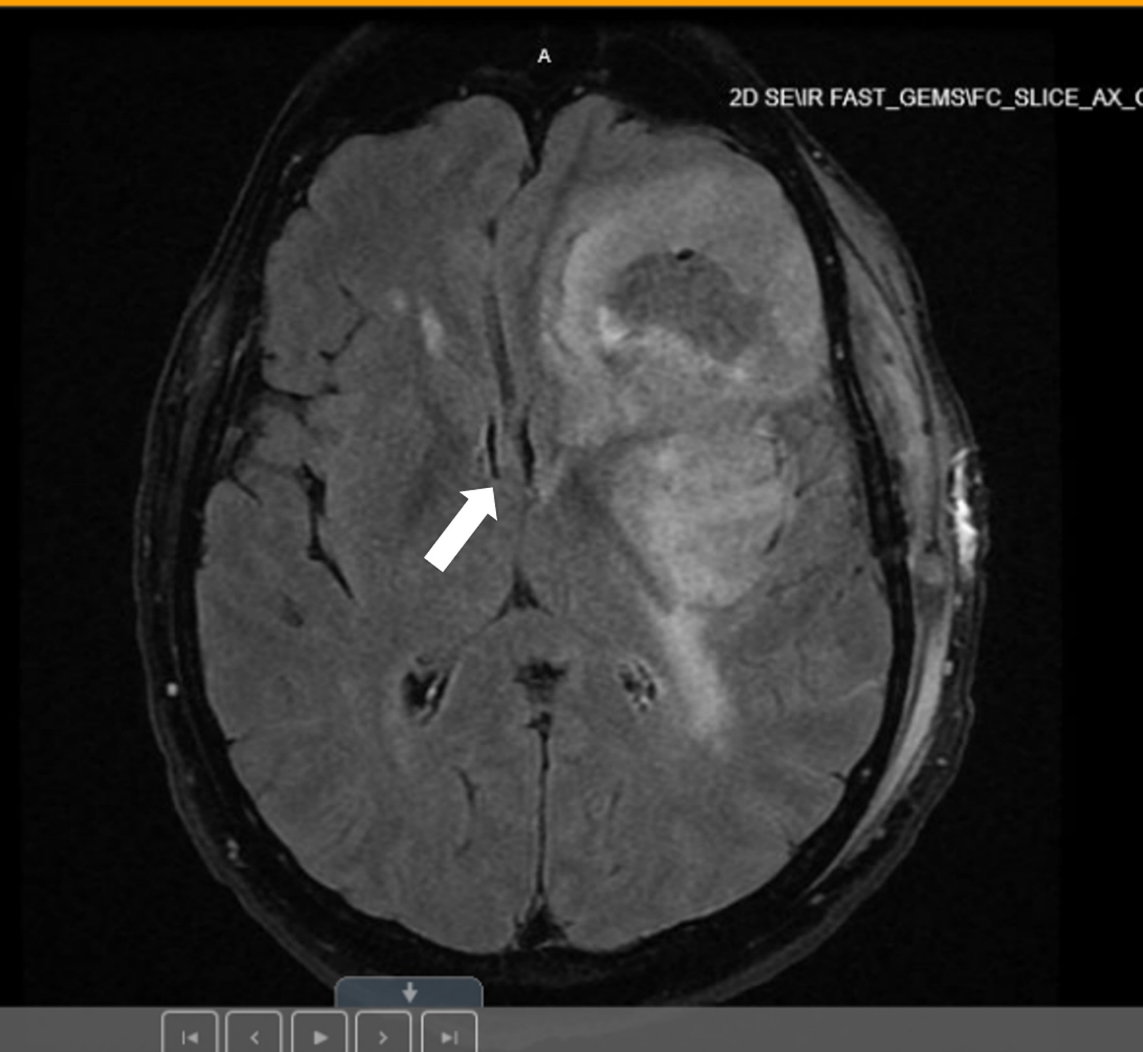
MRI of the brain demonstrates post partial resection of the large left frontotemporal tumor, with decreased peripheral contrast enhancement and decreased mass effect, resulting in diminished midline shift from left to right (denoted by a white arrow), now measuring 9 mm, compared to 13 mm preoperatively.
